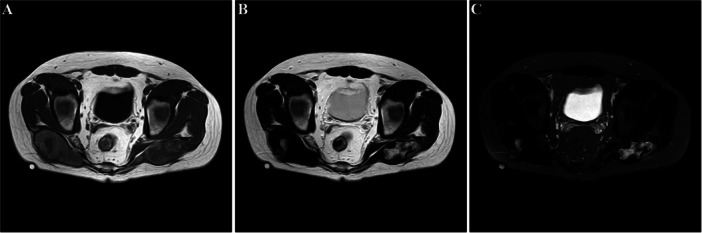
A: T1‐weighted MRI images demonstrate hyperintense signals in the peripheral regions and hypointense signal centrally within the lesions. B: T2‐weighted images reveal hypointensity peripherally and hyperintensity in the central regions. C: Fat‐suppressed T2‐weighted sequences reveal similar findings, with low signal intensity at the periphery and high signal intensity in the central areas of the lesions.
